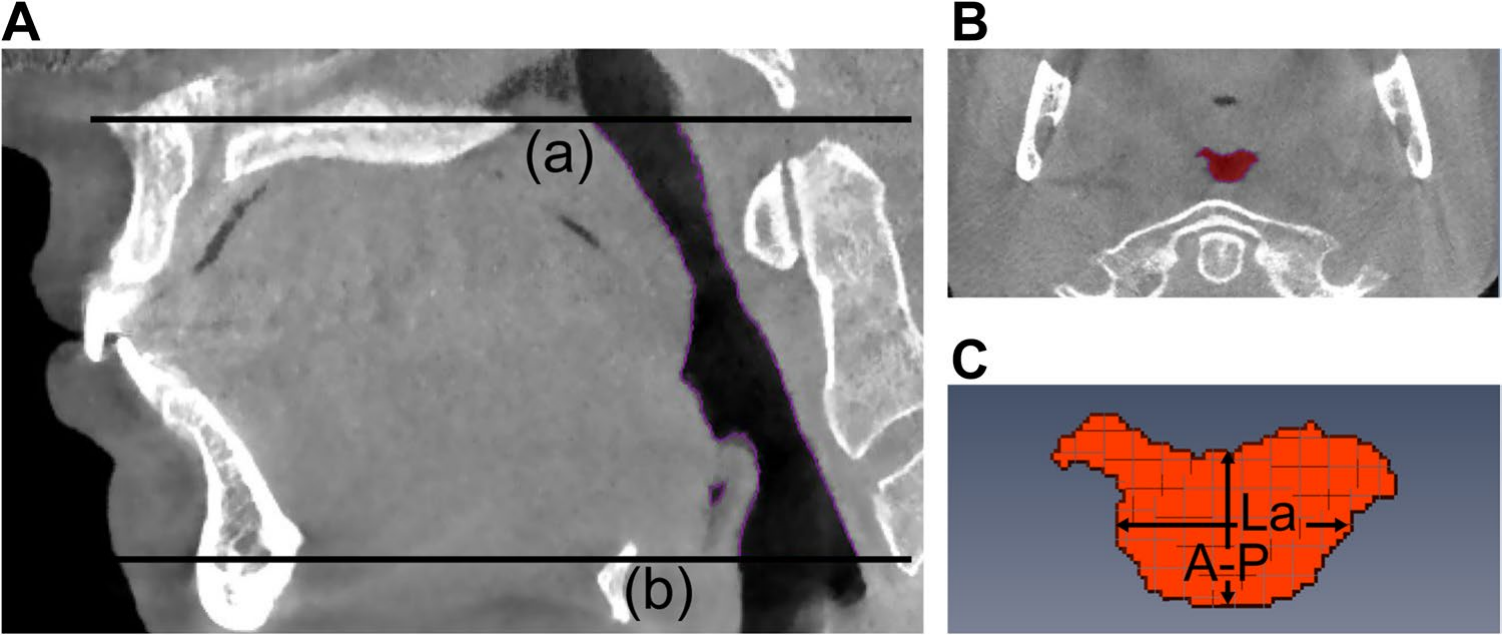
Measurements of the upper airway dimensions using cone beam computed tomography (CBCT) imaging. (A) The boundaries of the upper airway from the hard palate (a) to the base of the epiglottis (b) in the sagittal plane. (B) The location of the minimal cross-sectional area of the upper airway (CSAmin) in the axial plane. (C) The measurements of the anterior–posterior dimension (A-P) and lateral dimension (La) of the CSAmin
